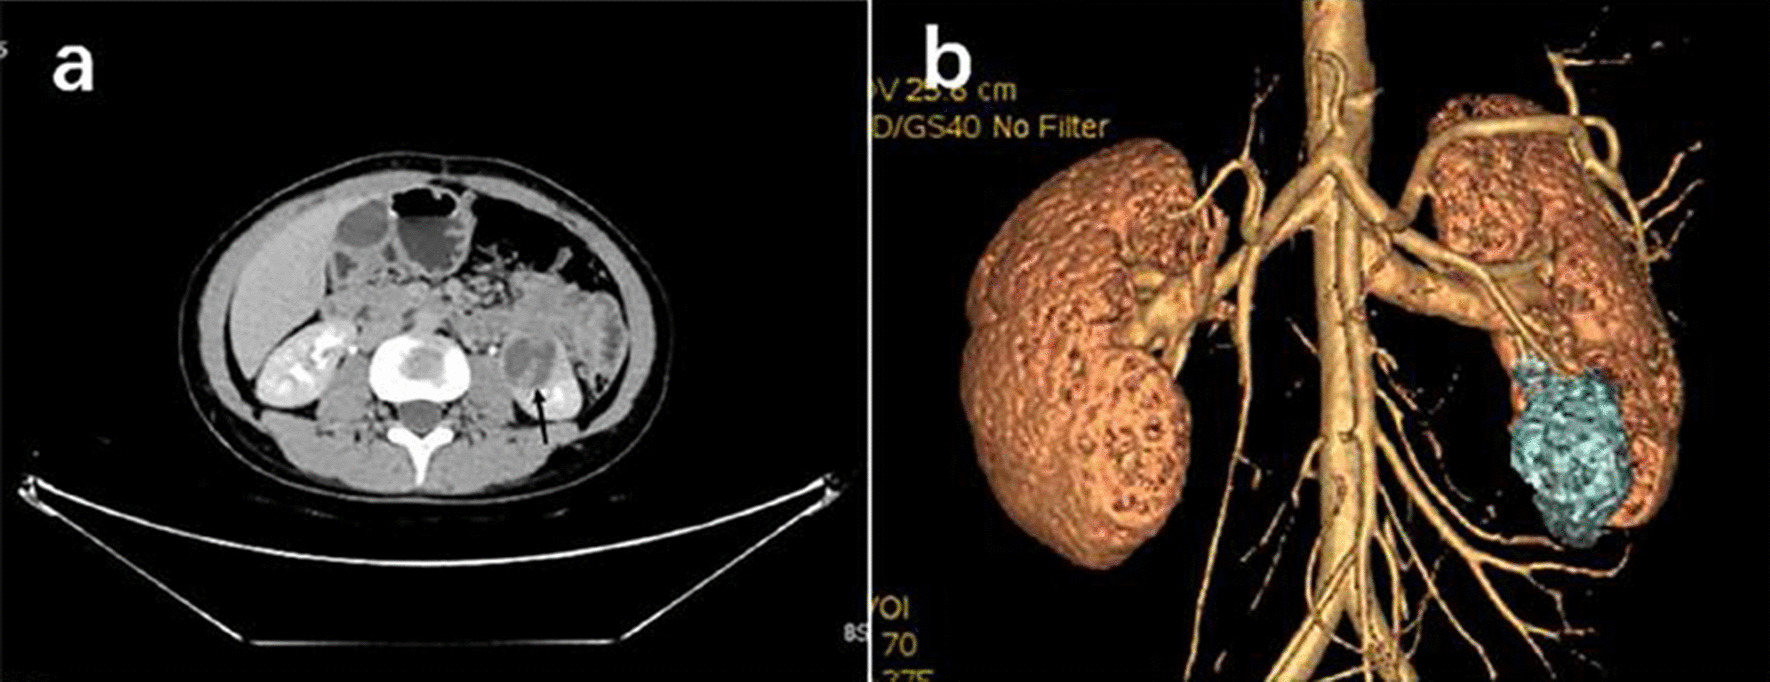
a Computerized tomography (CT)of the urinary system showed a heterogeneous mass in the left kidney(arrow). b Three-dimensional reconstruction of the kidney showed the lower 1/3 of the left kidney was occupied
